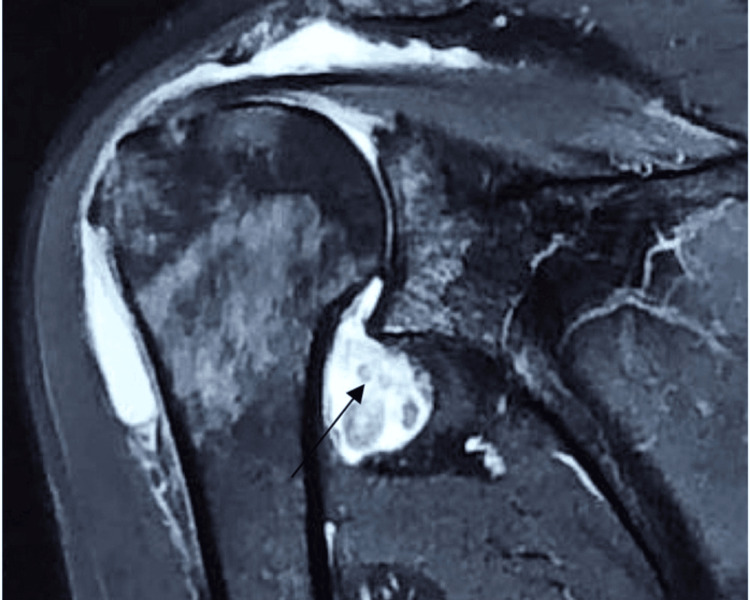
MRI demonstrating lobulated irregular intra-articular synovial thickening post-contrast enhancement
